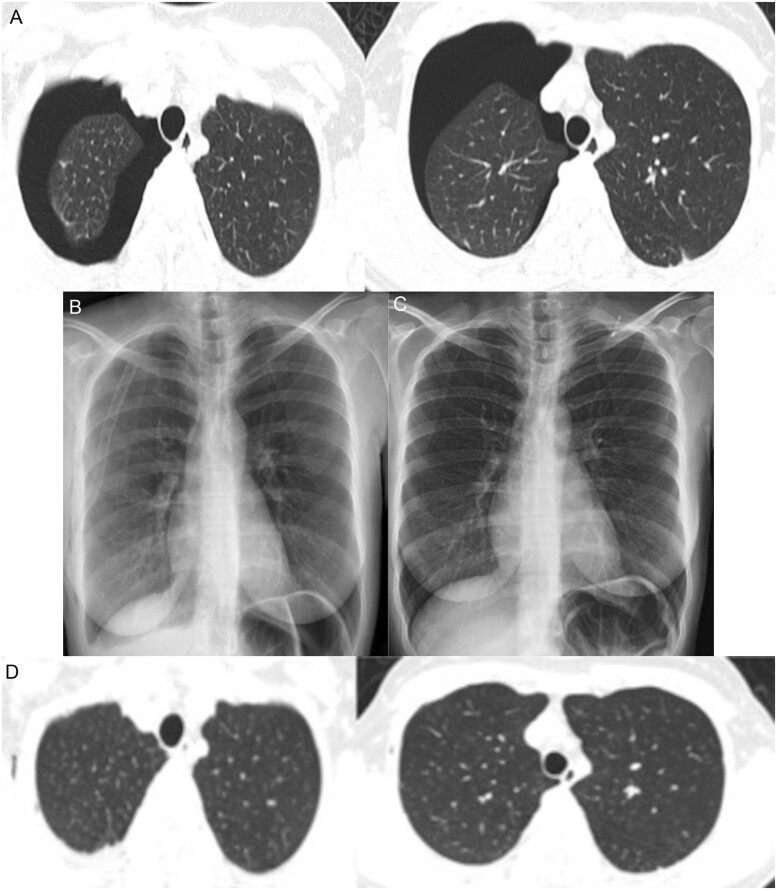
(A) Pneumothorax in the right lung on thorax computed tomography. No bulla or bleb was detected. (B) Lung expanded on X-ray image after chest tube thoracostomy. (C) Chest tube was removed on the fifth day of follow-up. (D) She was admitted to outpatient clinic 2 weeks after discharge, and her lungs observed expanded in both chest X-ray and thorax computed tomography.
